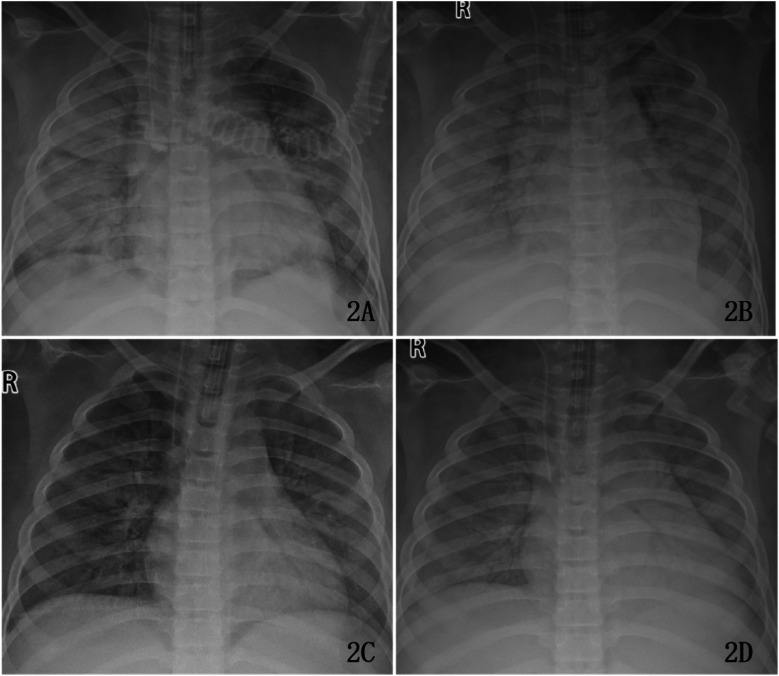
Changes in pulmonary infiltrates during ARDS treatment in the child. (A) Chest x-ray on day 3; (B) Chest x-ray on day 5; (C) Chest x-ray on day 6; (D) Chest x-ray on day 14.
